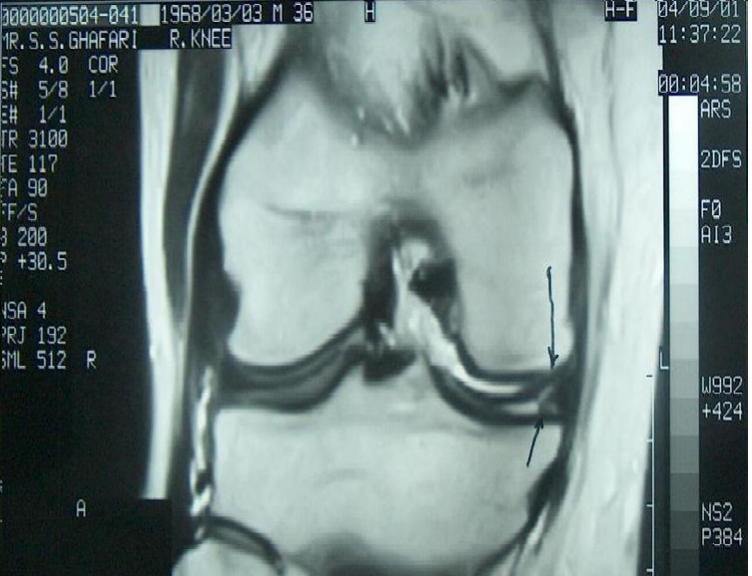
Magnified coronal T2-weighted MRI image of the right knee showing a medial meniscal tear.
